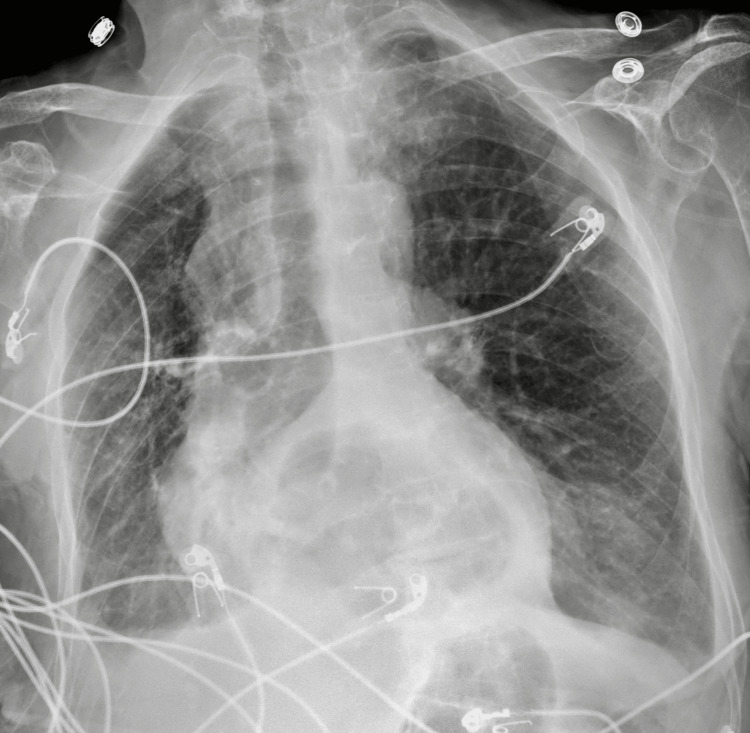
Portable anteroposterior chest radiograph Demonstrates mild interstitial pulmonary edema, right pleural effusion, and enlarged cardiomediastinal silhouette.
